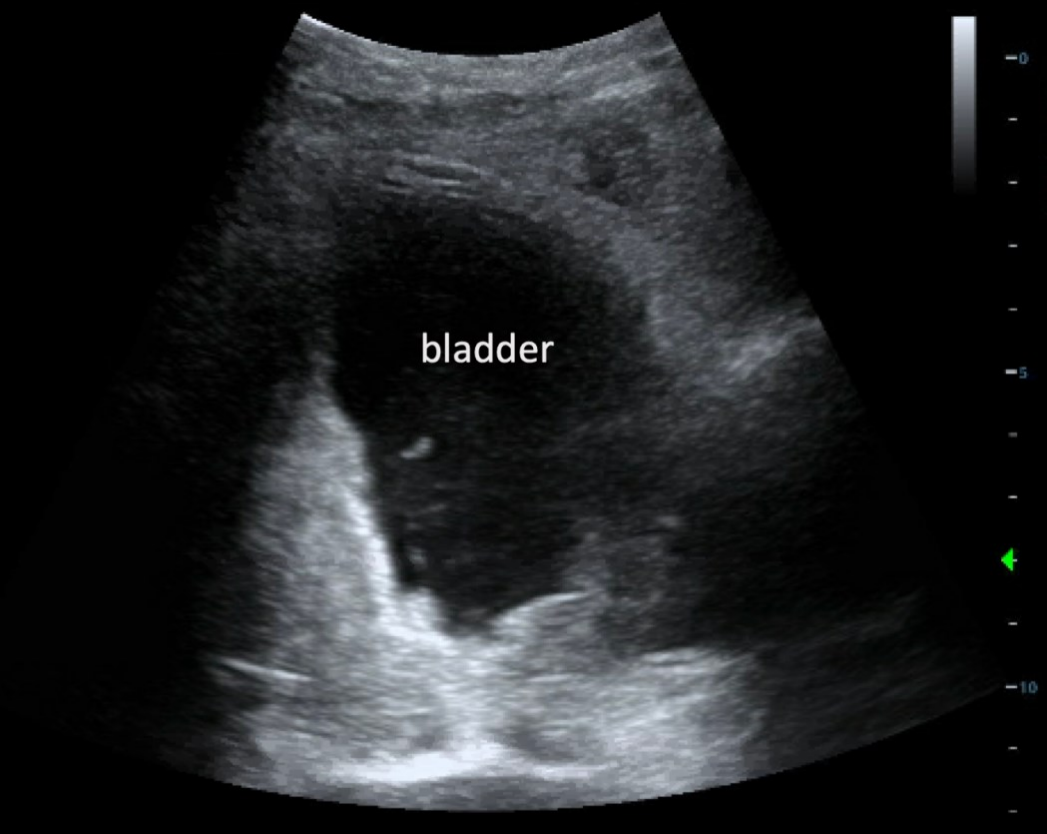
Point of care ultrasound in transverse plane demonstrating an irregularly-shaped bladder filled with anechoic contents and floating sediment.
